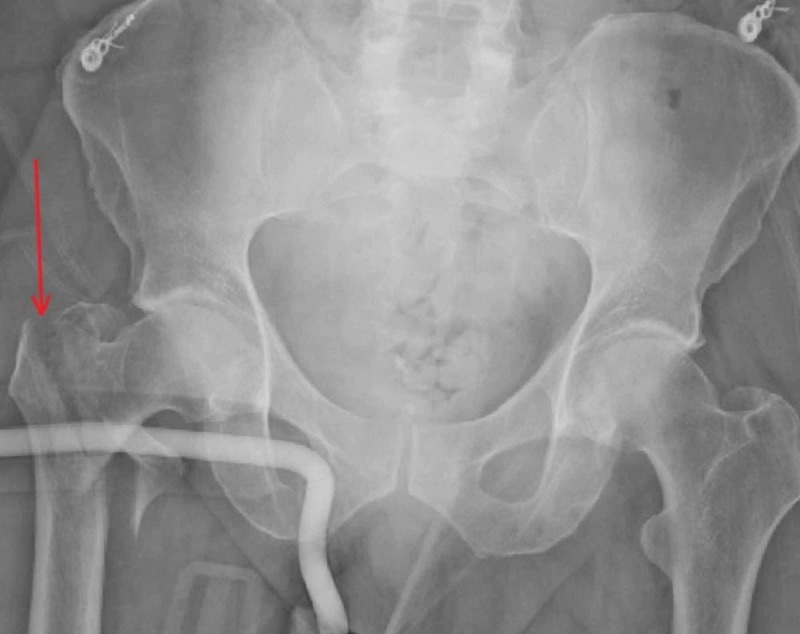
Pelvic X-ray of the displaced comminuted right intertrochanteric fracture (arrow)
